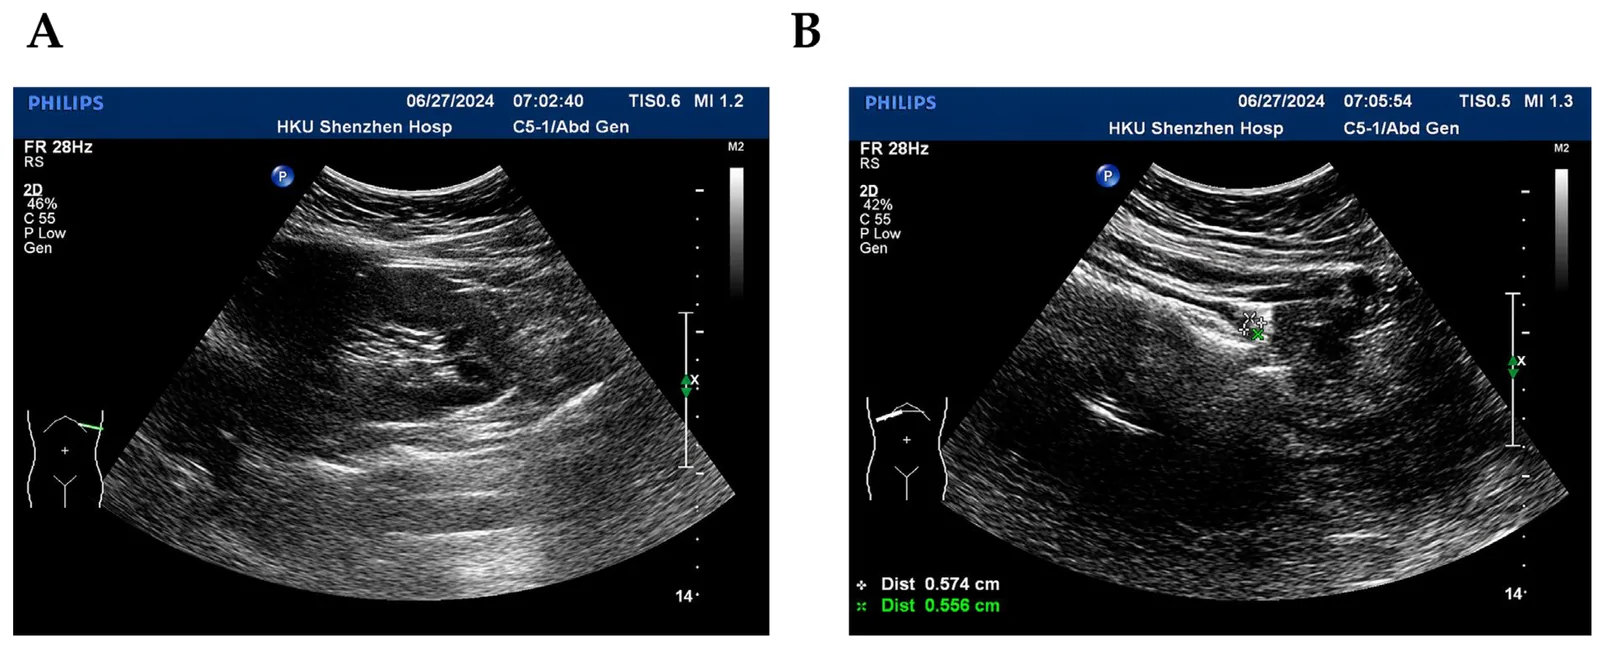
Urinary ultrasonography shows a right ureteral calculus with mild right hydronephrosis. The arrow indicates the 6 × 6 mm calculus located between the second and third anatomical constrictions of the right ureter. (A) Dorsal; (B) Abdomen.
